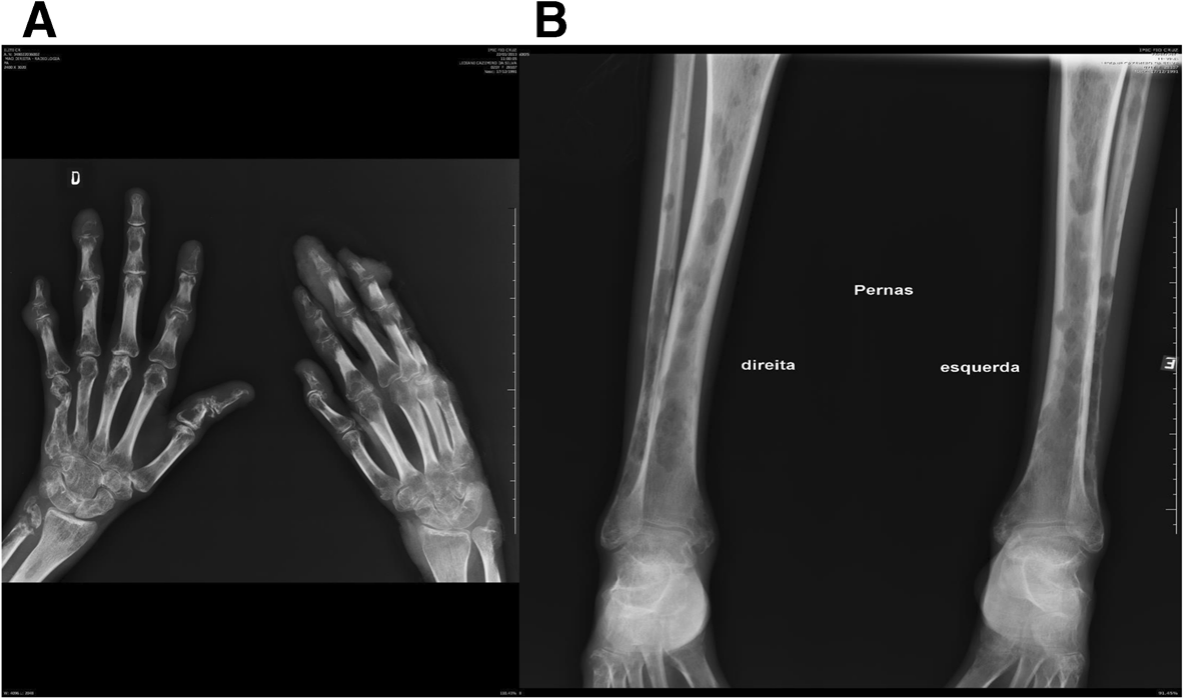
Plain radiographs of hands (A) and lower limbs (B) showing osteolytic lesions (on June 2012, admission to IPEC).
